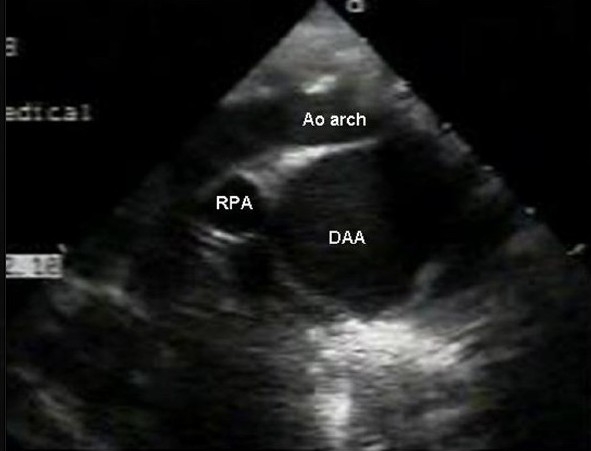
Two-dimensional echocardiography from modified suprasternal short axis view showing large ductal aneurysm with wide ampulla
